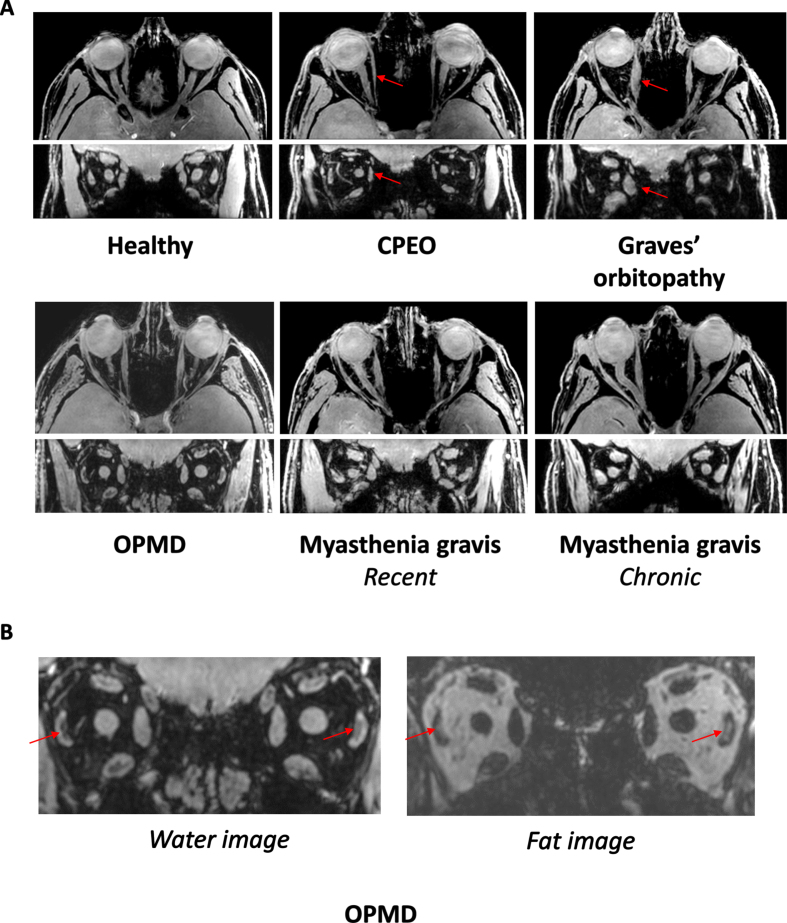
A. MRI scans from the orbit showing the extra-ocular muscles in a healthy control, a chronic progressive external orbitopathy (CPEO) patient, a Graves’ orbitopathy patient, an oculopharyngeal muscular dystrophy (OPMD) patient, a recent myasthenia gravis patient and a chronic myasthenia gravis patient. The axial and coronal water images are shown from a chemical shift based water-fat separation gradient echo scan, a technique to separately image and quantify water and fat. The red arrows indicate the atrophic medial rectus muscle in the CPEO patient and the swollen medial rectus muscle in the Graves’ orbitopathy patient. B. Example of fat replacement of the lateral rectus muscles in an OPMD patient as depicted by the red arrows. On the left the coronal water image and on the right the coronal fat image are shown.
